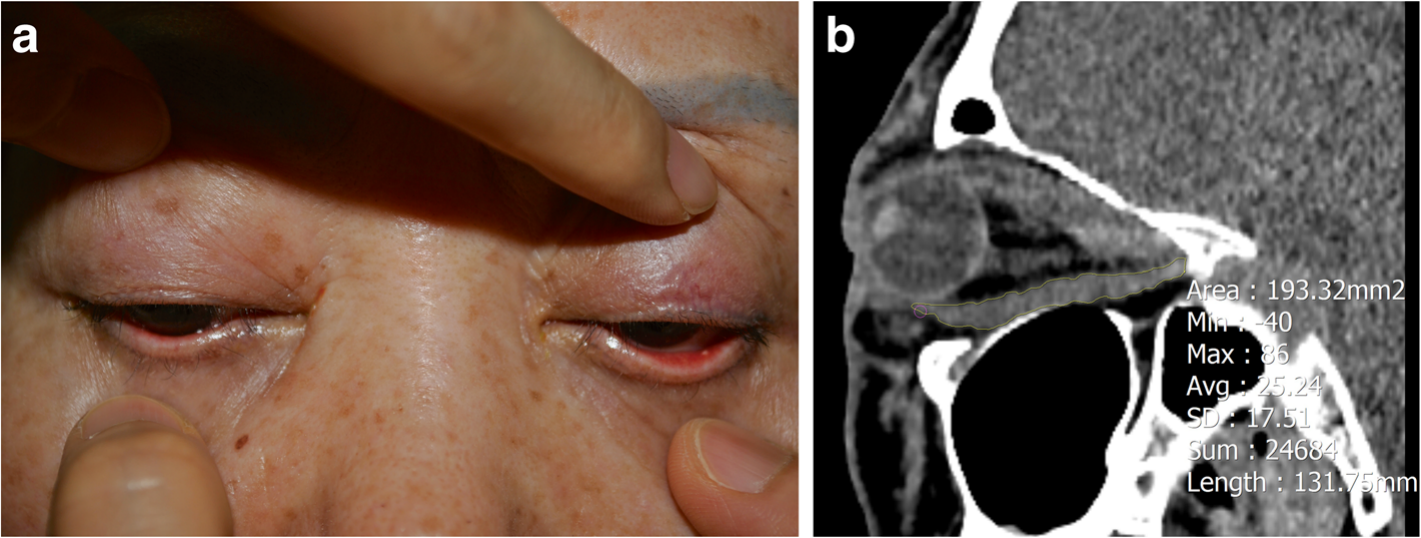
A Bell’s phenomenon is assessed by gently lifting each upper lid manually while the patient’s eyes are closed. The examination shows absent BP in both eyes. B Measurement of cross-sectional areas of inferior rectus muscle on sagittal CT images
